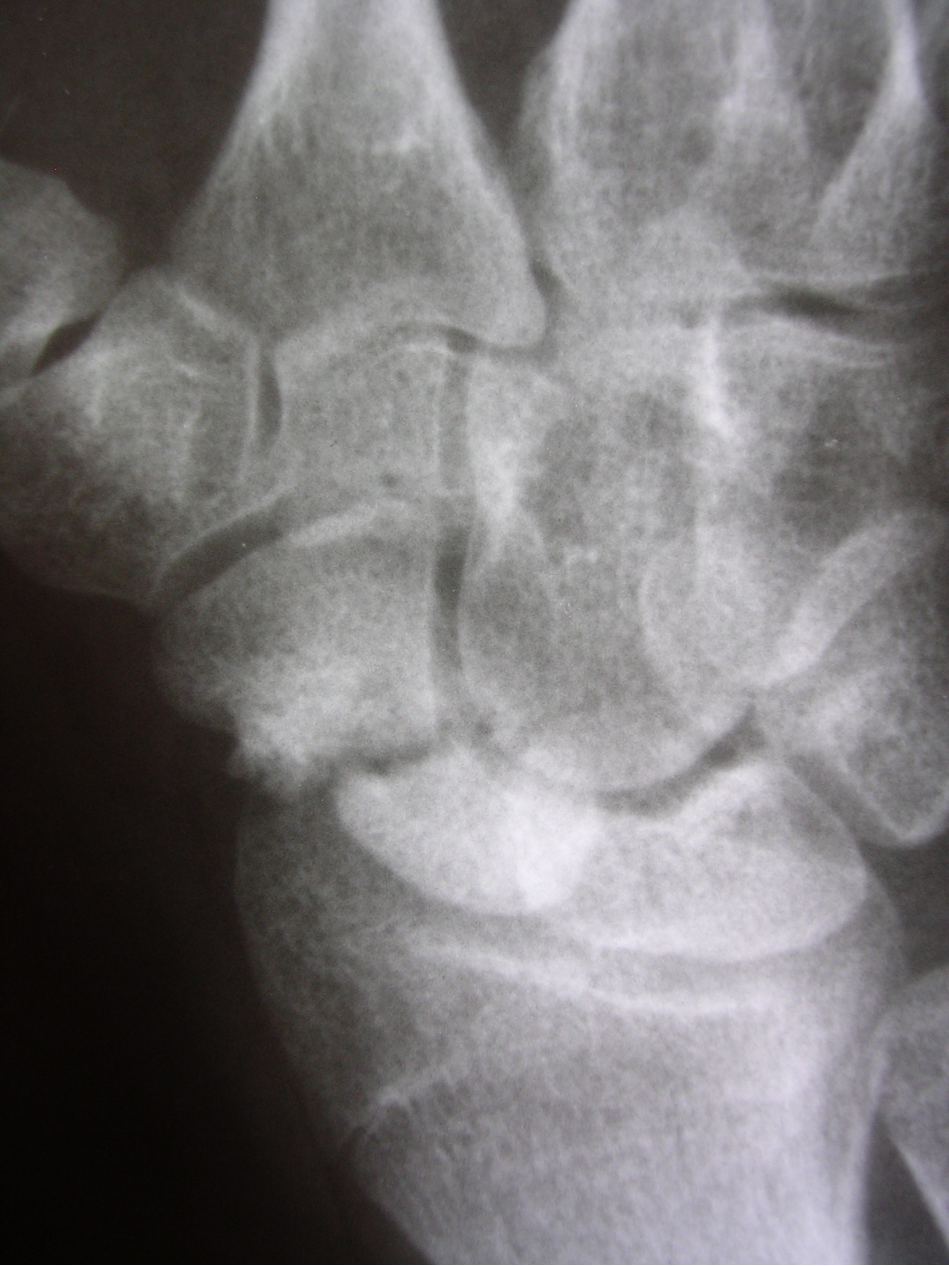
Scaphoid non-union (SNU) in patient number 3, a preoperative radiograph.
